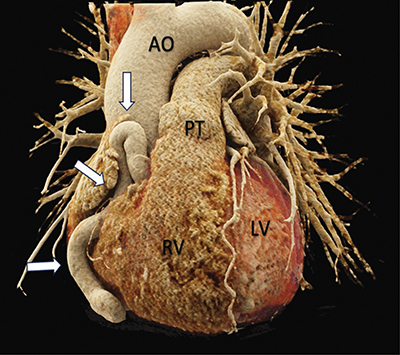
3D rendered cardiac CT-angiography reveals a tortuous dilation of the right coronary artery (white arrows). Front view. *Source:* AO = Aorta, PT = Pulmonary Trunk, RV = Right ventricle, LV = Left Ventricle.
